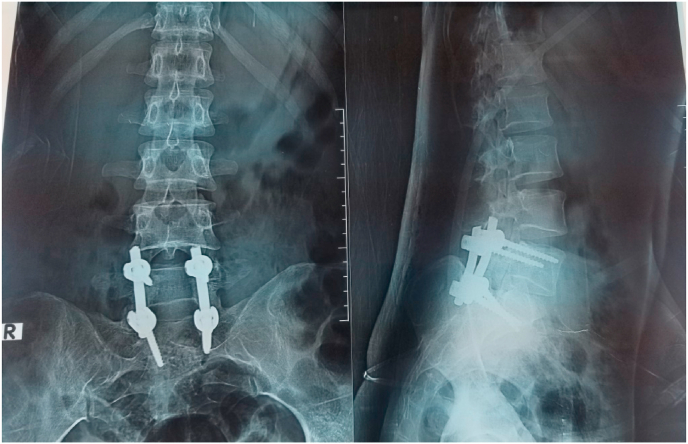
Postoperative X-ray of the patient (fixed with screw and rods).
